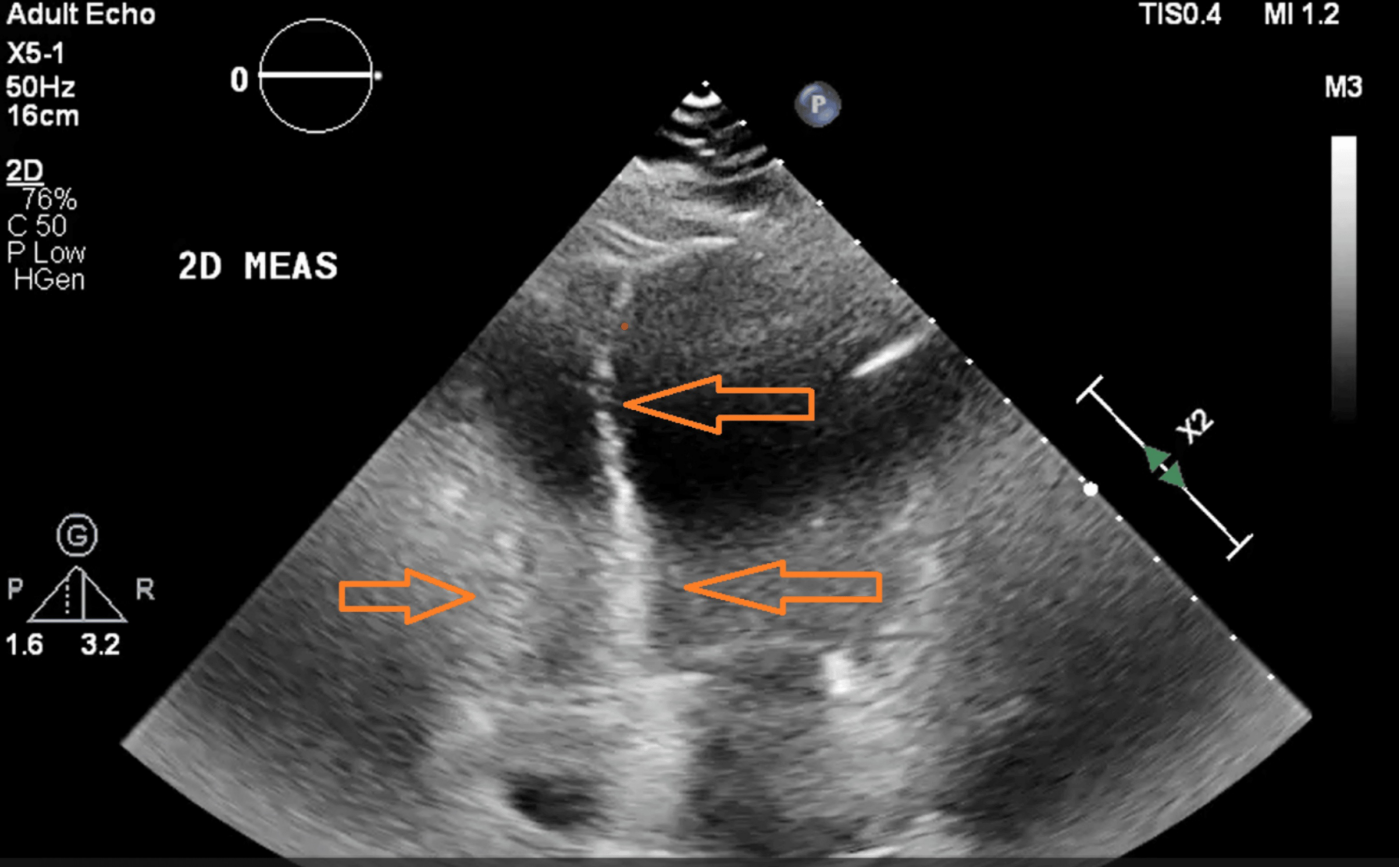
Four-chamber view demonstrating a moderately dilated right ventricle and right ventricle hypertrophy of 9.4 mm with an elevated right ventricular systolic pressure at 40-50 mmHg, consistent with pulmonary hypertension. POCUS expedited recognition and referral prior to formal echocardiography POCUS: point-of-care ultrasound
